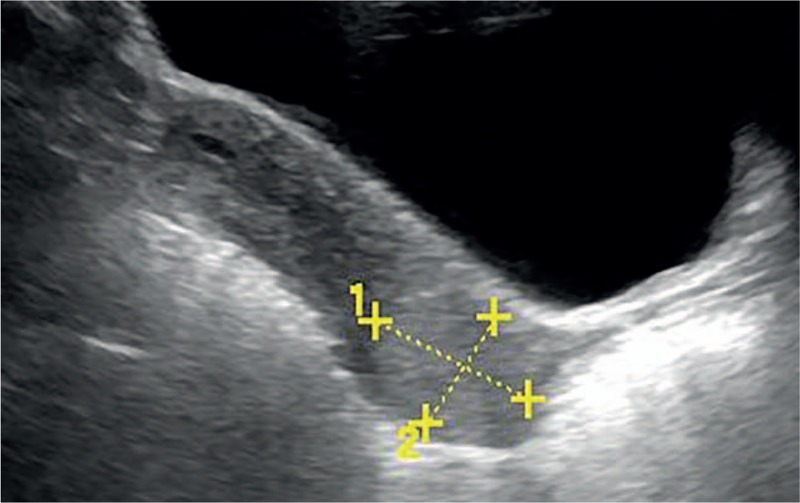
Transabdominal ultrasound imaging showing a well-circumscribed, strongly echogenic lesion in the cervix with endometrial cavity minimal fluid. The uterus was normal size. The endometrial thickness was 0.5 cm. No obvious abnormality was found in the double accessories. The lesion corresponded to an ETT on pathological examination.
